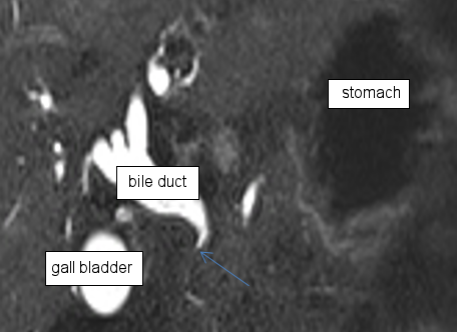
MRI with widened bile duct and the ectopic orifice into the pyloric region
